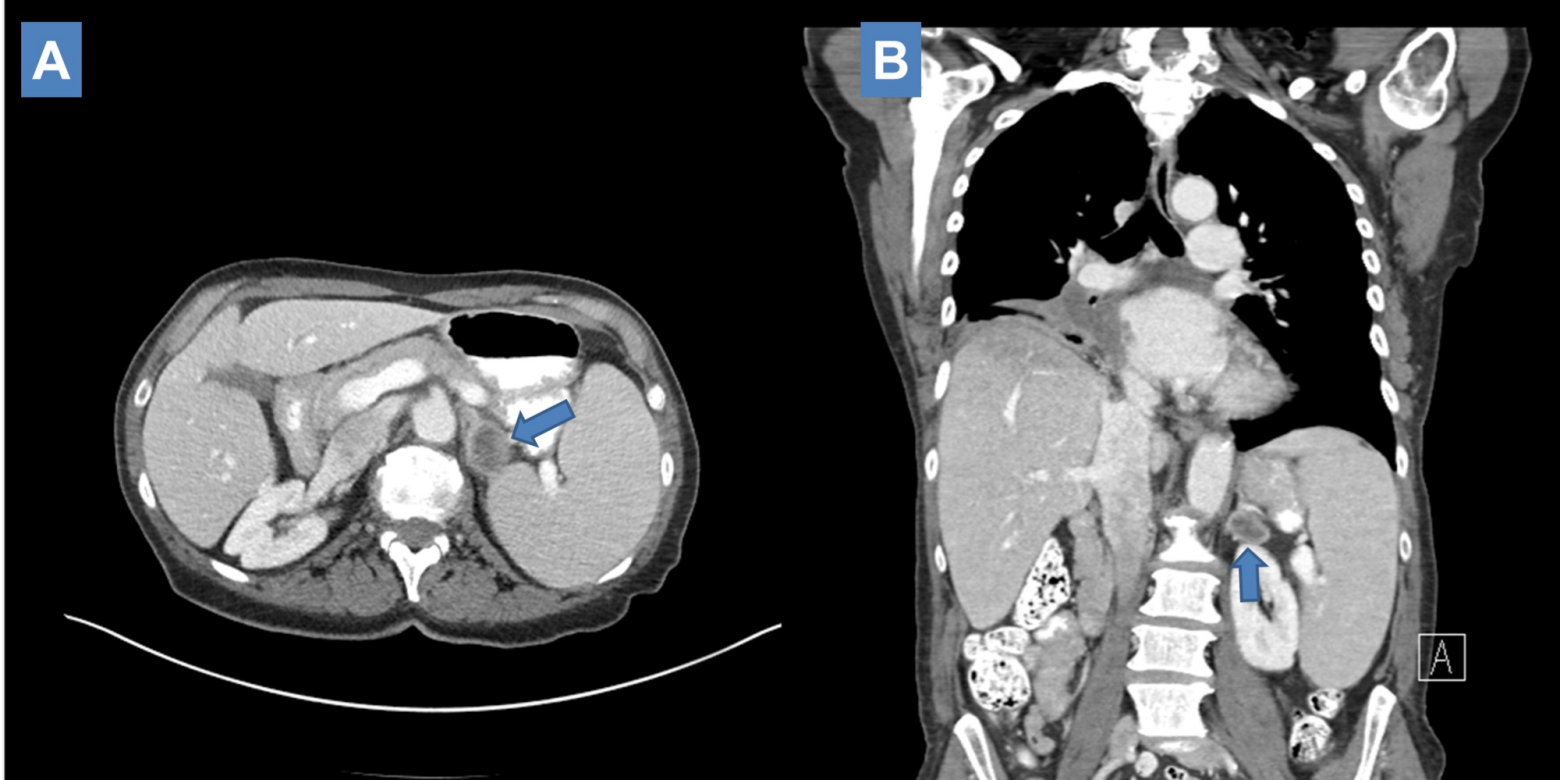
Initial post definitive treatment computed tomography (CT) scan disclosing new left adrenal metastasis (2.8 cm x 2.4 cm). (A: Axial view; B: Coronal view).
